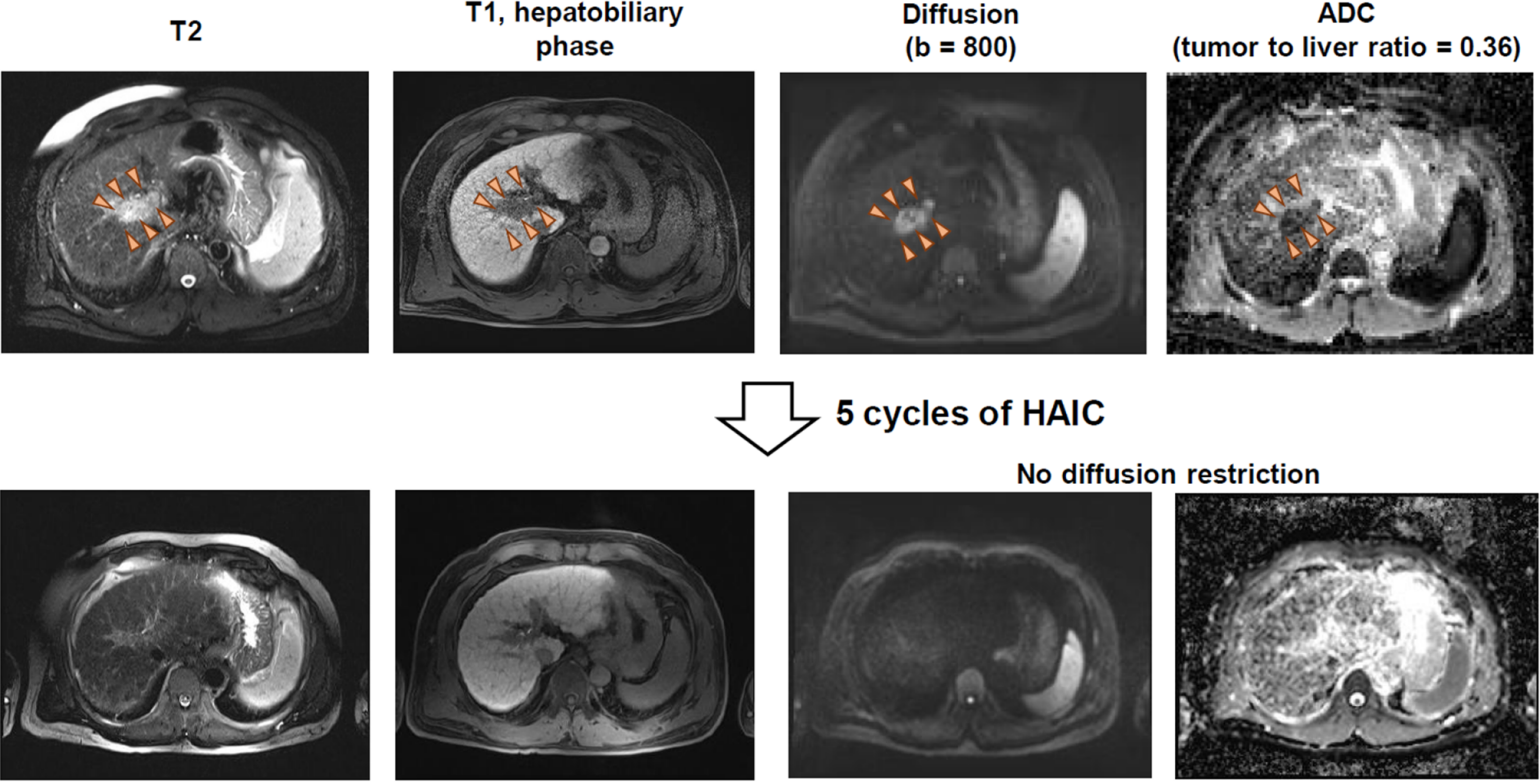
MR imaging of a patient having HCC with strong diffusion restriction and good response to HAIC.
